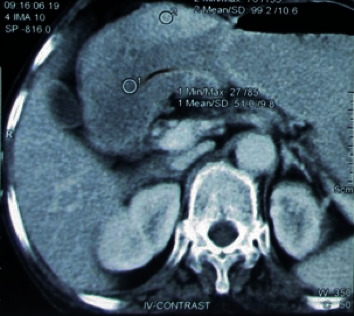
Axial computed tomography image of the abdomen showing a thickened wall of the stomach at the body and pyloric end.
